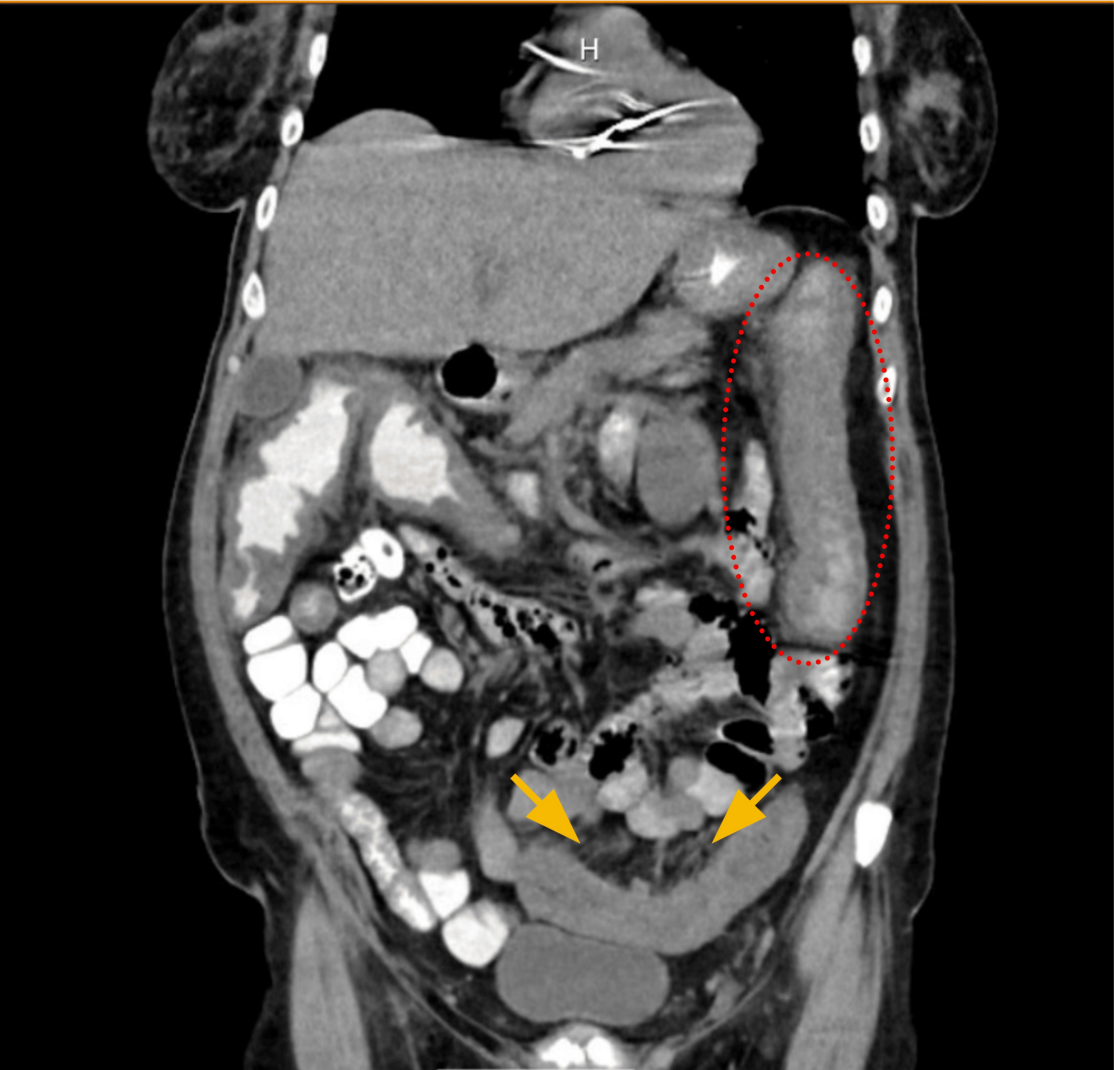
CT of the abdomen shows diffuse colonic wall thickening and peri-colonic fat stranding Yellow arrows indicate areas of peri-colonic fat stranding, while the red dotted circle highlights colonic thickening in the descending colon.
